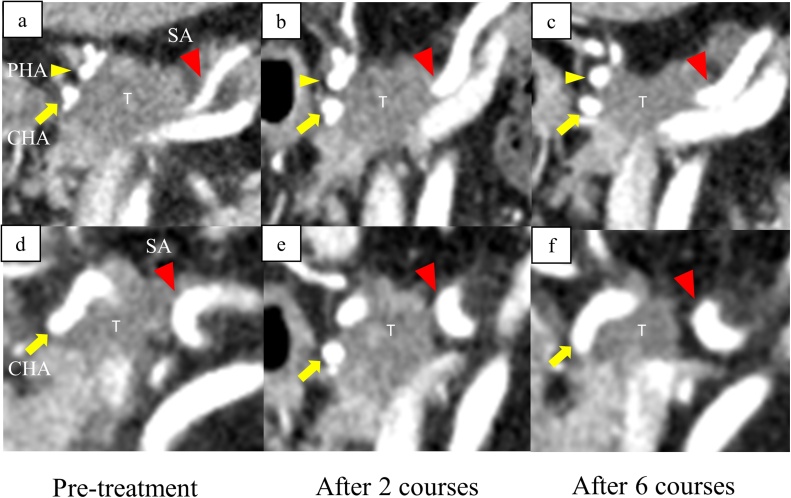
Images from pre- and post-chemotherapy coronal enhanced computed tomography. T: tumor; yellow arrow: CHA; yellow arrowhead: PHA; red arrowhead: SA. a, d) Before GnP chemotherapy. The tumor is in wide contact with the CHA, PHA, and SA. b, e) After 2 cycles of GnP. Distance between the tumor and SA is slightly increased. c, f) After 6 cycles of GnP. The tumor has shrunk, and tumor contact with the SA has disappeared, but contacts with the CHA and PHA remain.
